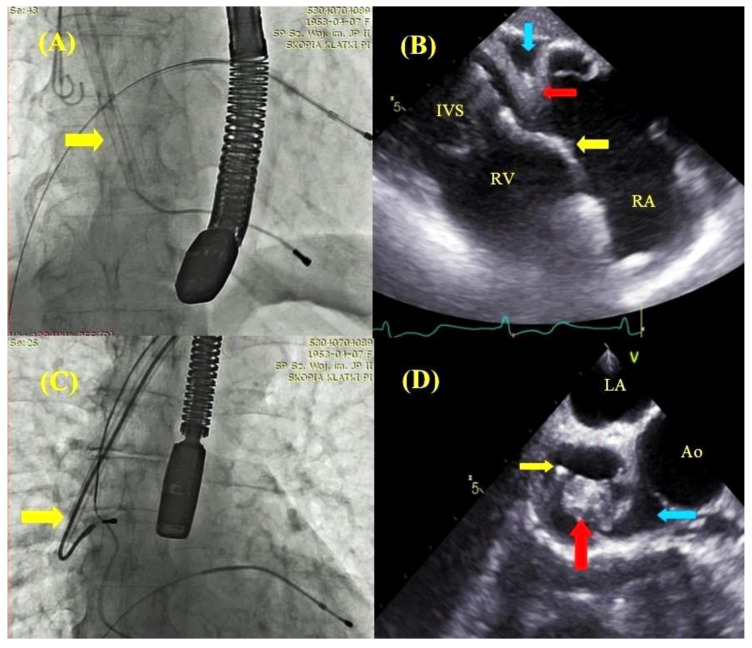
Comparison of fluoroscopic and echocardiographic images during lead extraction maneuvers. The moment of ventricular lead extracting; the yellow arrow points to the Byrd dilator slipped over the lead (A). TEE—transgastric view—the moment of ventricular lead extraction (A) with pulling on the right ventricular (RV) wall (red arrow), hyperechogenic thickened lead adhered to the endocardium (yellow arrow), the separation of pericardial layers corresponding to pseudo-cardiac tamponade (blue arrow) (B). The moment of atrial lead extraction; the yellow arrow points to the Byrd dilator slipped over the lead (C). TEE—mid-esophageal view; simultaneous atrial lead extraction (yellow arrow) (C), right atrial appendage prolapse into the atrial lumen (red arrow) and the separation of pericardial layers corresponding to pseudo-cardiac tamponade (blue arrow) (D).
